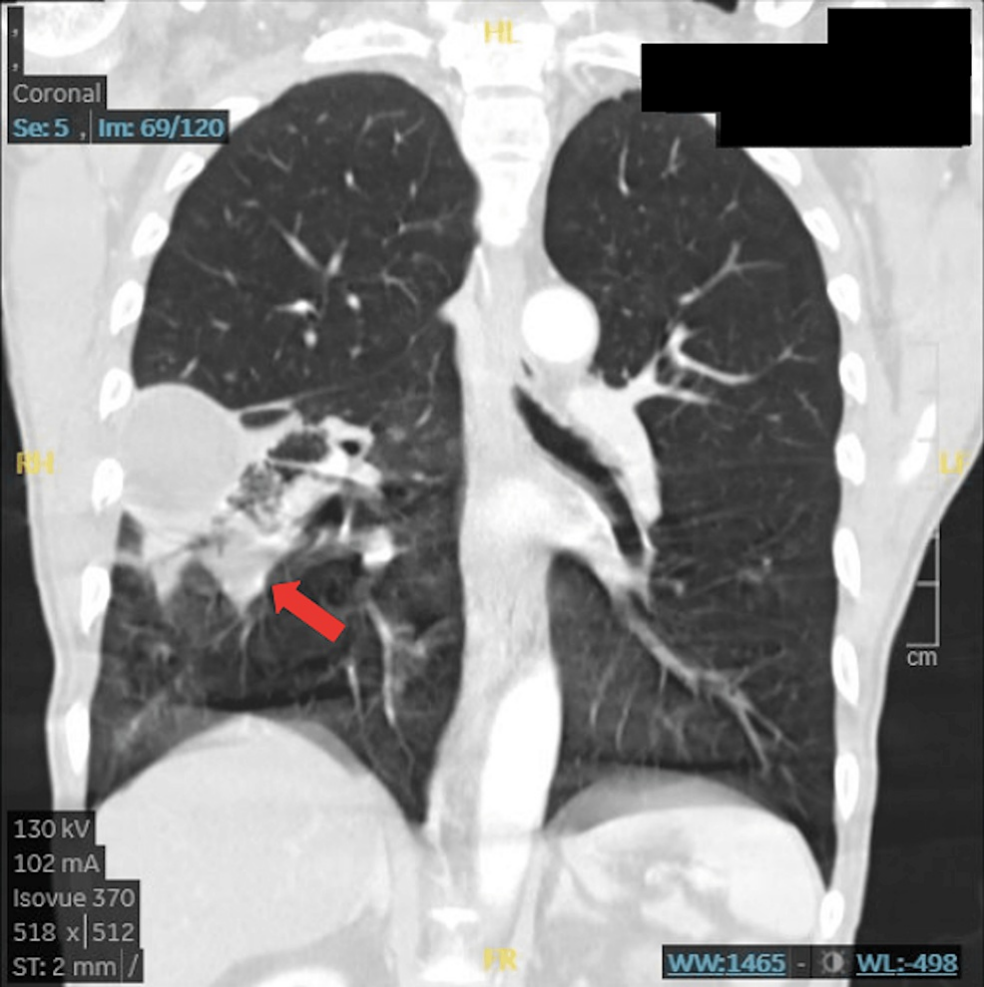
Sagittal CT scan of the complicated abscess. The red arrow points to the area of loculation.
